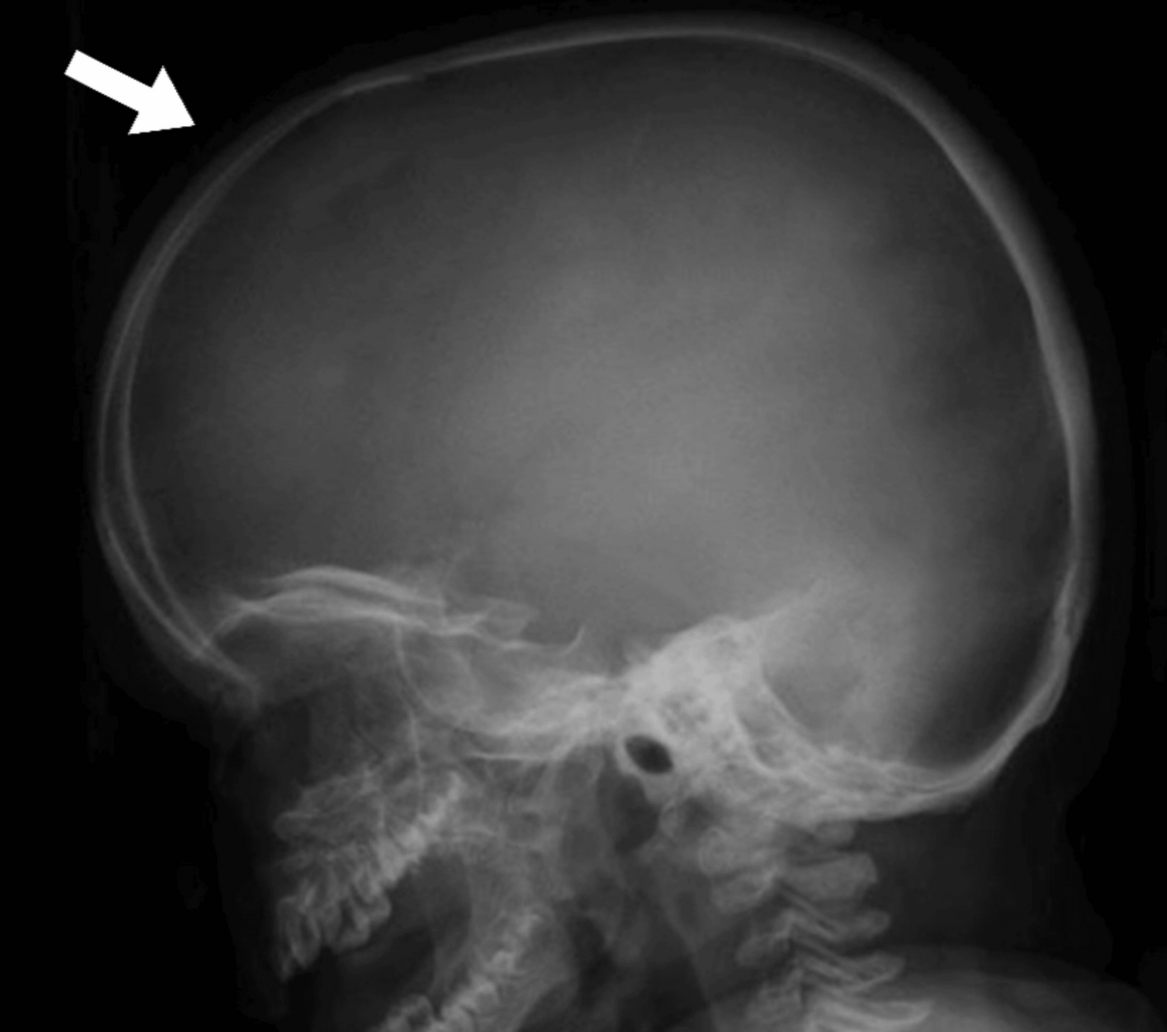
Skull X-ray showing macrocephaly, with the arrow highlighting the skull
